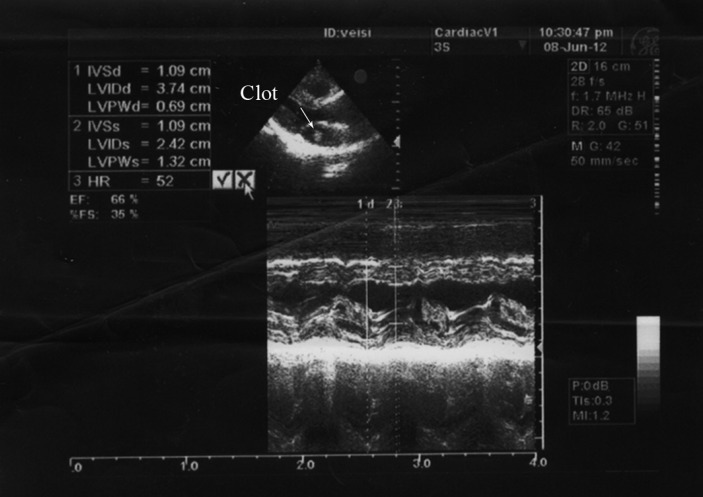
Transthoracic echocardiography in M mode and parasternal view, showing a clot (arrow) attached to the residual resected interatrial septum. No pericardial effusion is seen
